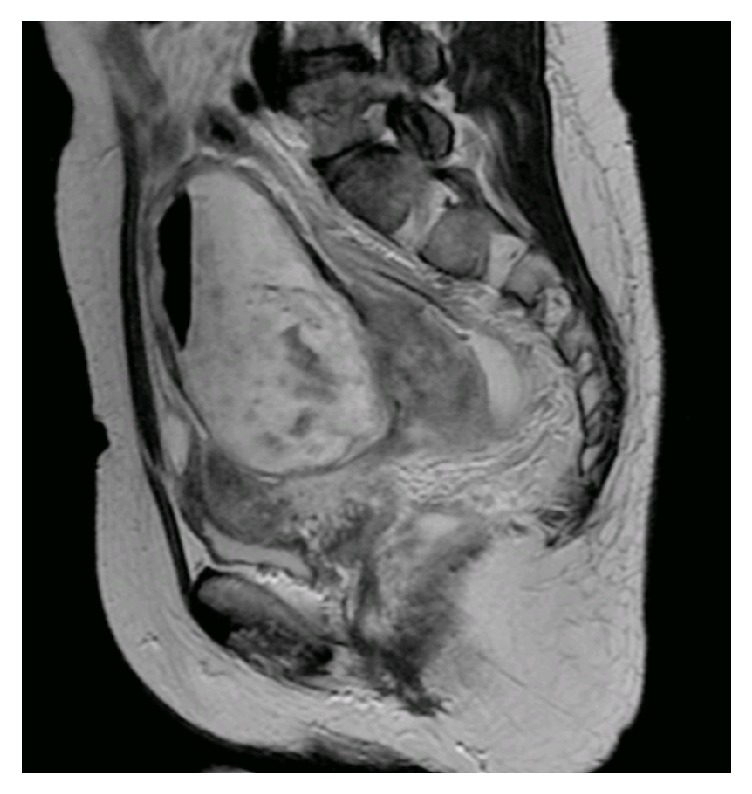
Abdominopelvic magnetic resonance imaging shows a complex mass containing a pocket of air in the pouch of Douglas.
